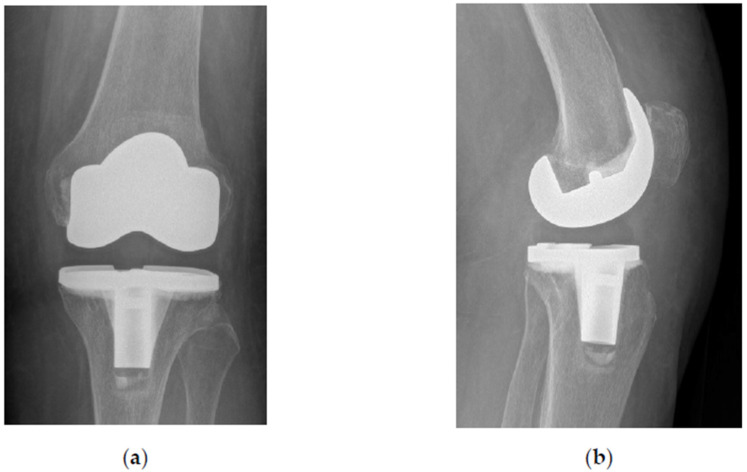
Standard X-rays of the left knee after total knee arthroplasty (TKA). (a) Anteroposterior view; (b) lateral view.
